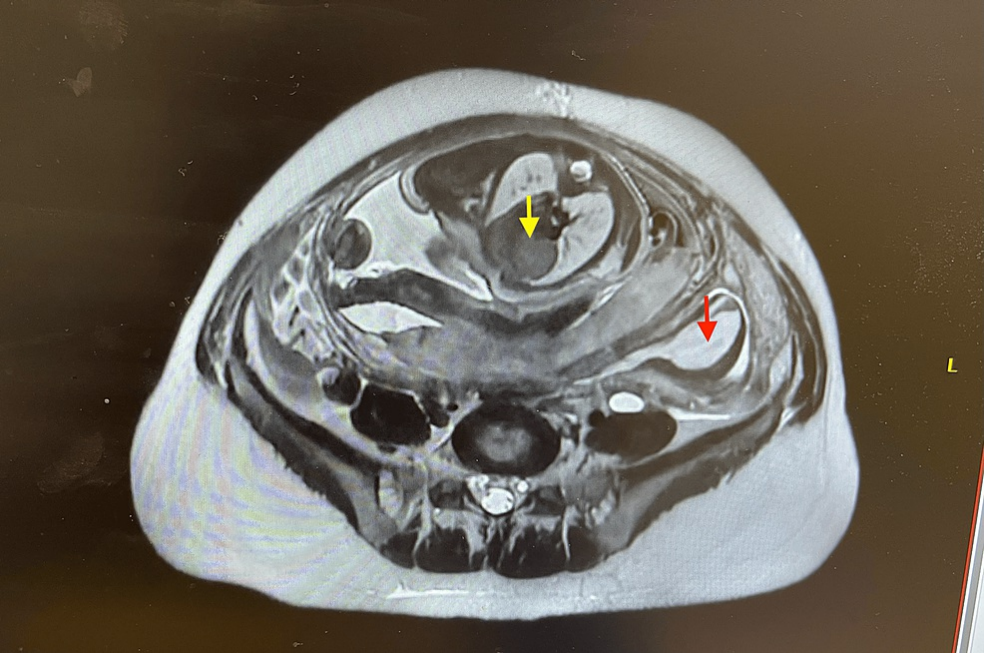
Transverse view MRI of the abdomen and pelvis. The image describes a transverse view magnetic resonance image (MRI) of the patient's pelvis showing a complex left adnexal cyst (marked with a red arrow) adjacent to the uterus, which harbors the fetus, marked with a yellow arrow.
